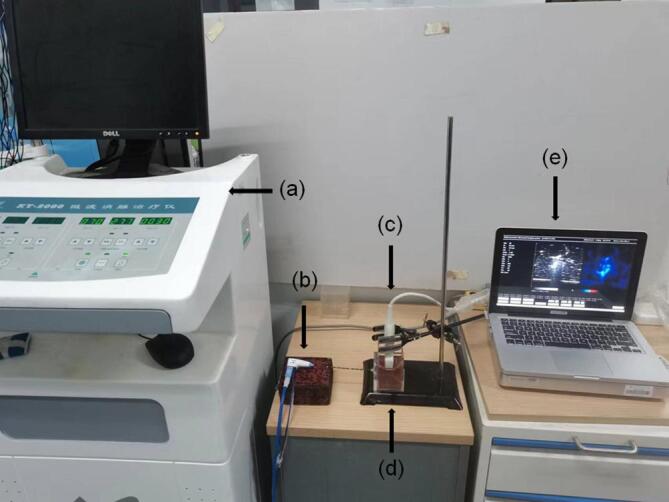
Experimental setup for microwave ablation and ultrasound backscattered signal acquisition. (a) microwave ablation device; (b) microwave ablation needle; (c) linear-array transducer; (d) polycarbonate box; (e) ultrasound scanner.
